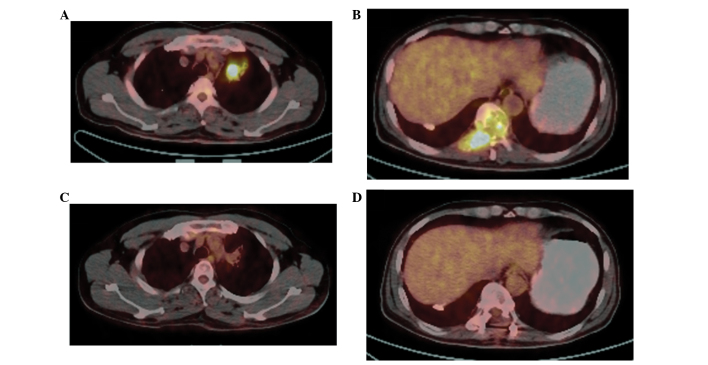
(A and B) PET-CT scan showed an abnormal mass at the level of T9 and the left upper lobe of the lung. (C and D) Twenty-four months after treatment, PET-CT scan showed inactivation of the neoplasm in the left upper lobe and T9 vertebra and (C) a decrease in the left upper lobe mass in the short axis. PET-CT, positron emission tomography-computed tomography.
